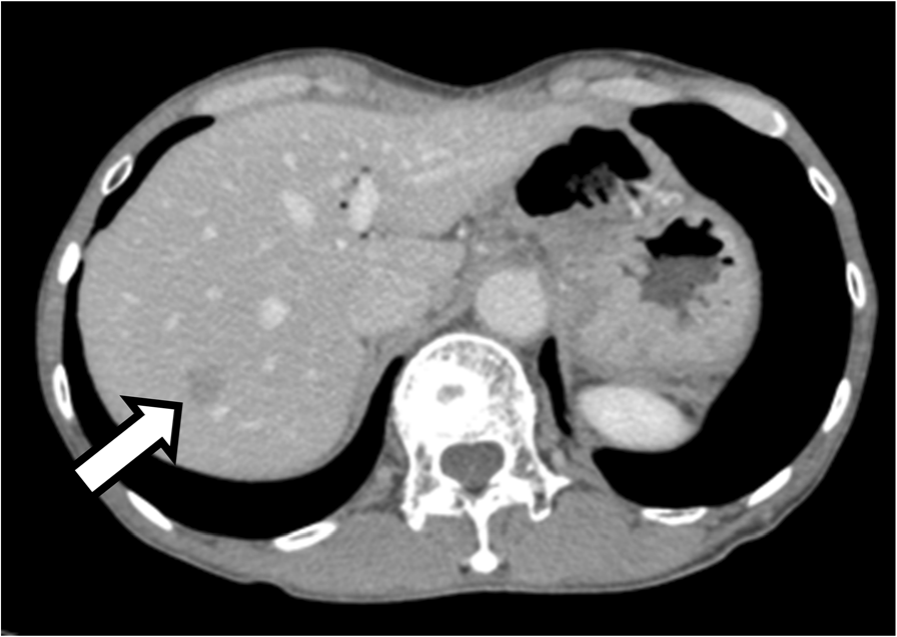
Episode 2: hepatic metastasis. Twenty-four months after the pancreatectomy. Contrast-enhanced computed tomography (CT) scan showed a solitary 1.5-cm hypovascular nodule in segment 7 of the liver (arrow)
